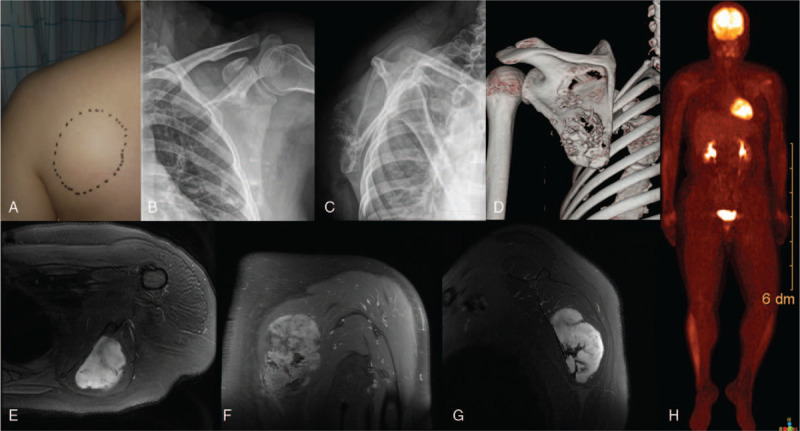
A 41-year-old woman presented with a palpable mass in the scapular area (A) and a bone lesion at the scapula on plain radiography (B and C). A lobulating contoured heterogeneous enhancing mass with internal calcifications was identified in the left infrascapularis muscle with adjacent scapula destruction (D–G). No evidence of distant metastasis can be found (H).
